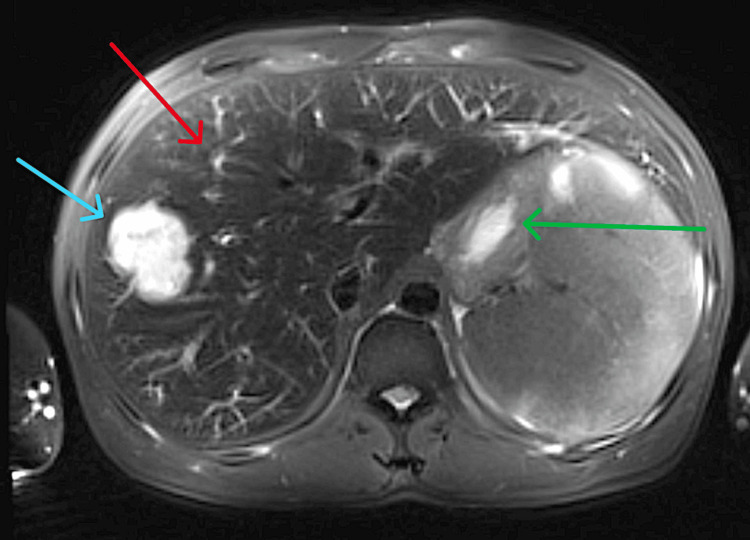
The MRI of the abdomen revealed hepatomegaly with hemosiderosis (red arrow), hepatic hemangioma (blue arrow), and splenomegaly with hemorrhagic infarcts (green arrow). MRI: magnetic resonance imaging
